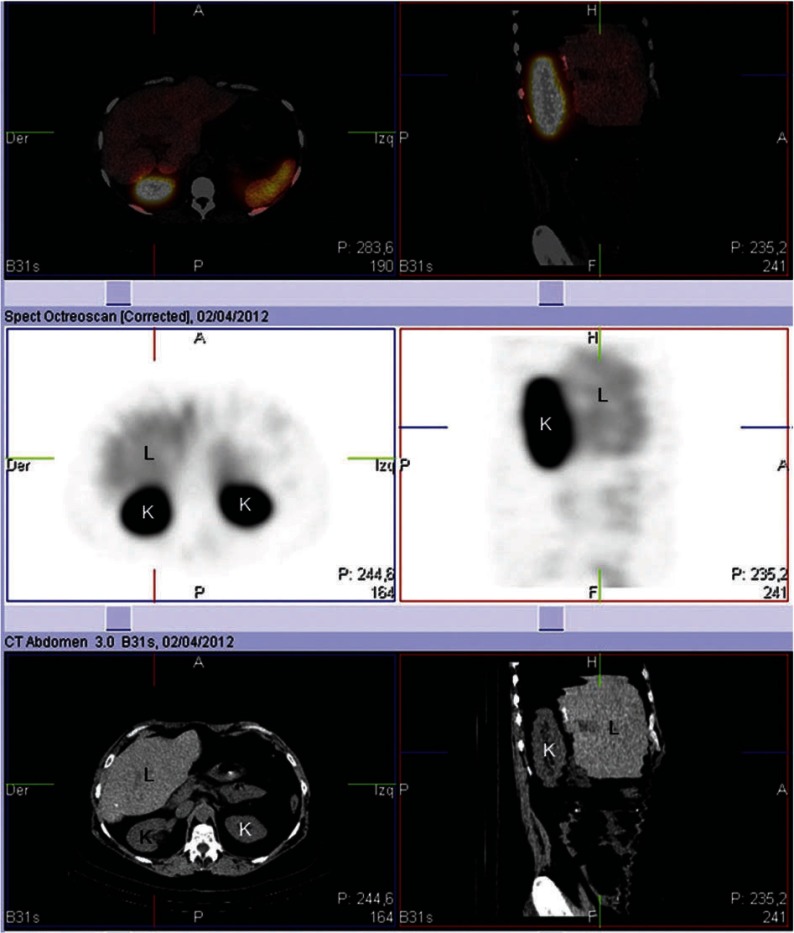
Abdominal Computed Tomography, Whole-Body Scintigraphy and Hybrid Study Abdominal computed tomography (CT) (lower images), whole-body scintigraphy (central images) and hybrid study [single photon emission computed tomography (SPECT)-(CT)] (upper images) with indium-111-octreotide showing a typical distribution of the radiotracer with preferential visualization of the liver, the spleen and the renal system without pathological accumulation activity. L: liver, K: kidney.
